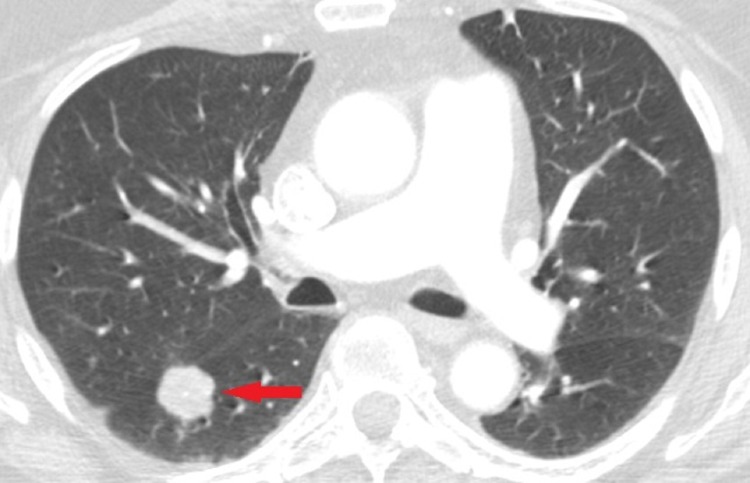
CT chest showing a benign right lower lung mass CT: computed tomography
